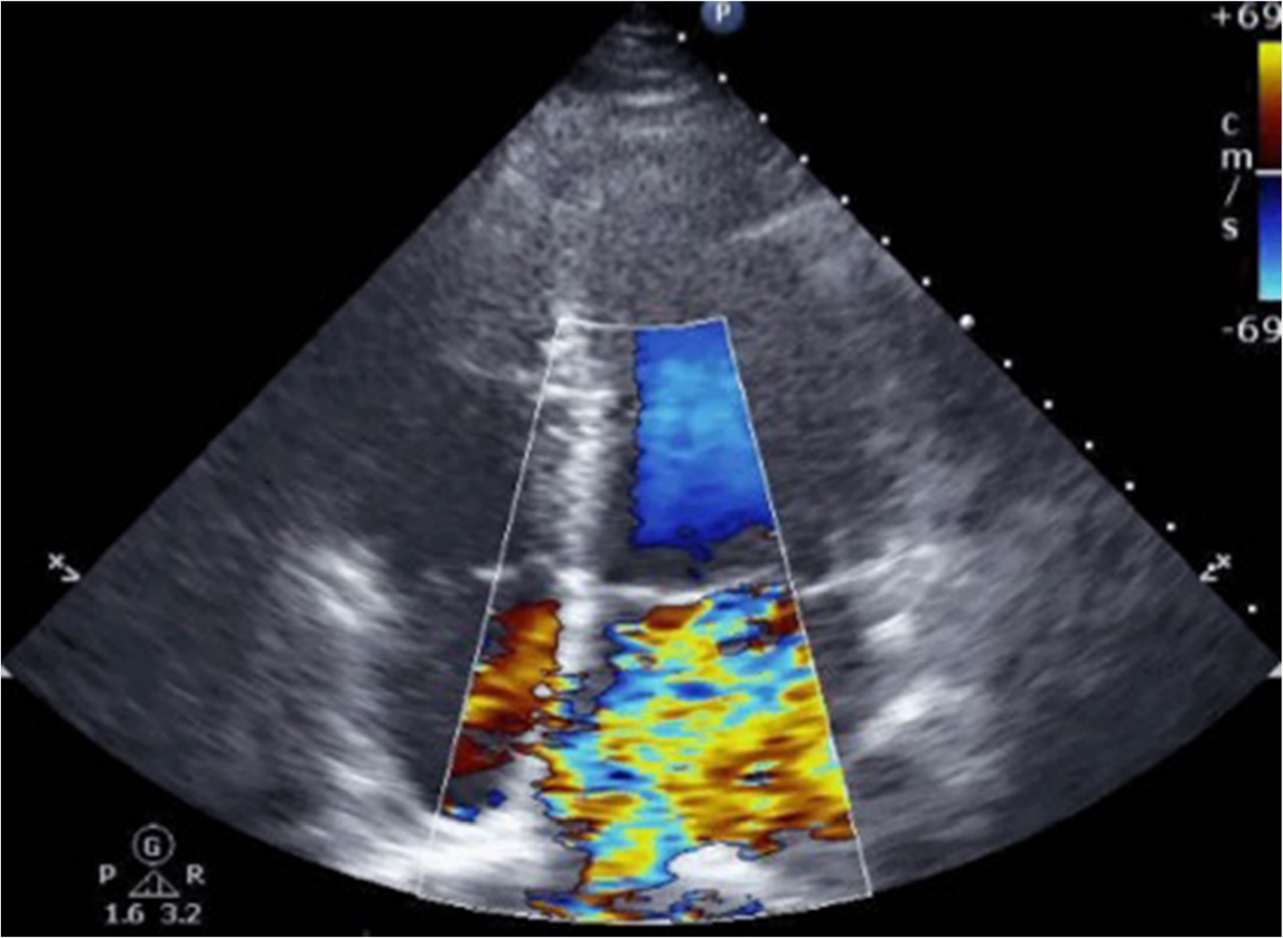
Transthoracic echocardiography with Doppler revealed a severe mitral valve regurgitation from the apical four chamber view
